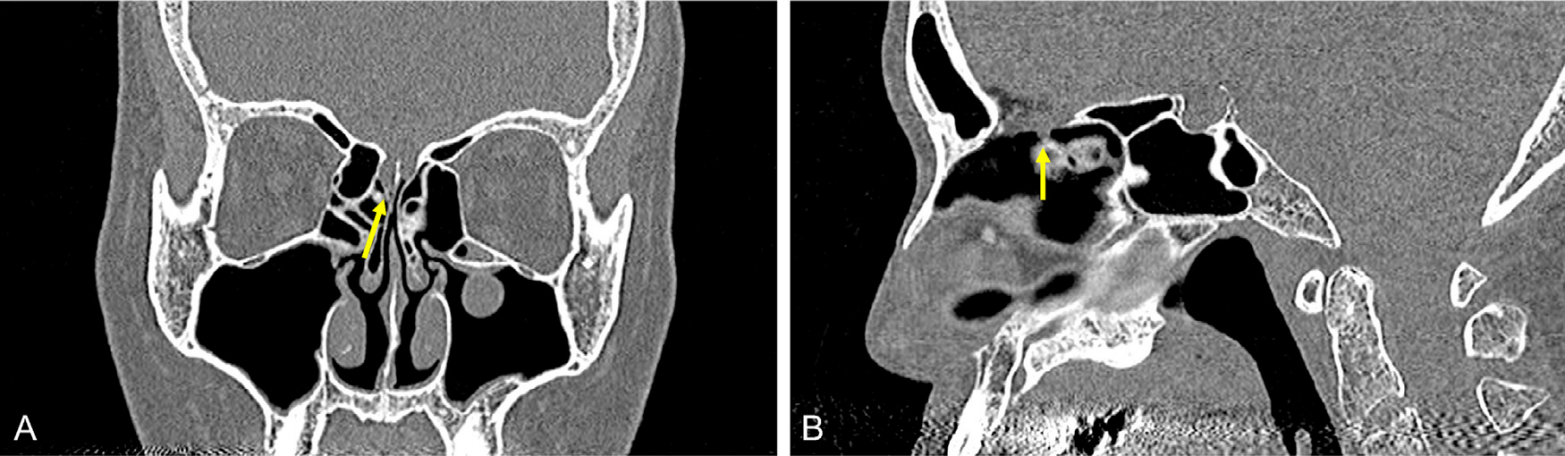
Reconstruction of the initial CT with a 0.5-mm slice. The coronal image (A) and sagittal image (B) of the CT reveal a protrusion of soft density from the right ethmoid cribriform plate toward the nasal cavity (arrow).
